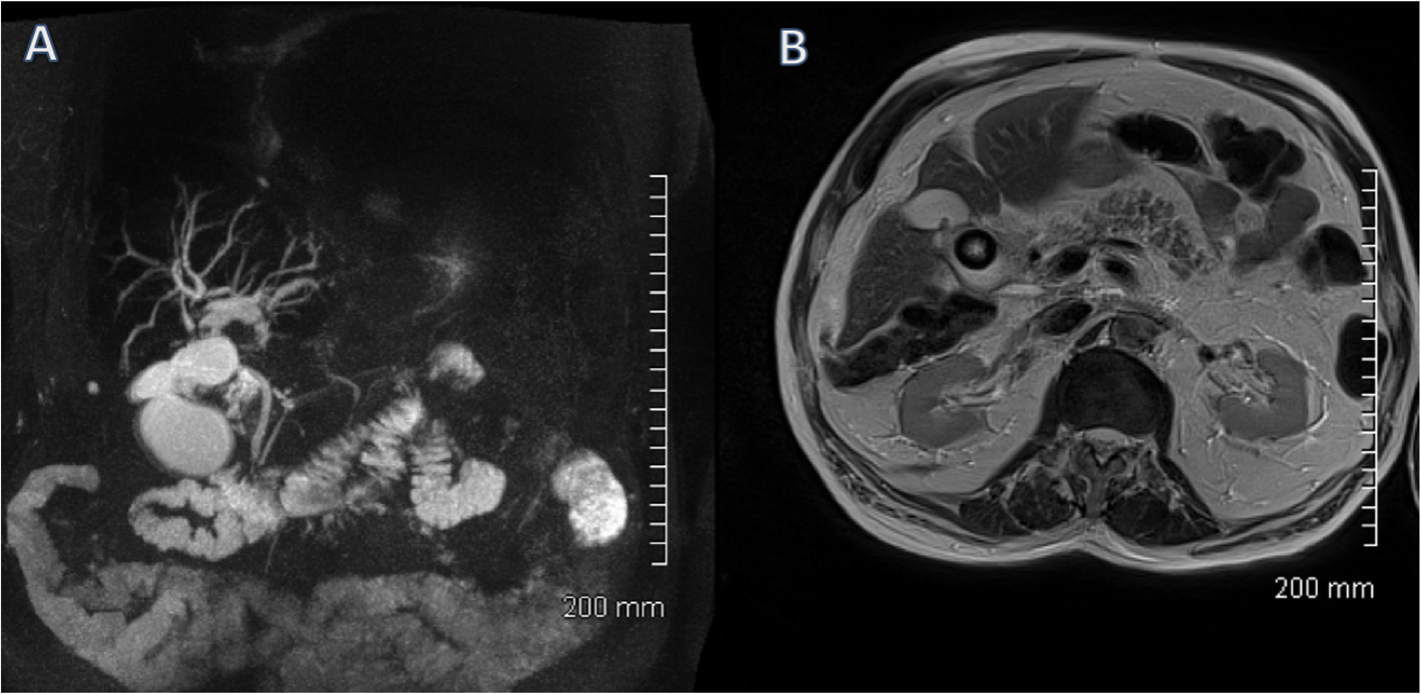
a Maximum intensity projection (MIP). b Axial T2-weighted MRCP image of the biliary tree. The gallbladder is distended with extensive intrahepatic biliary duct dilatation. The common bile duct is normal in calibre. Appearances are consistent with Mirizzi syndrome, with a stone in Hartmann’s pouch of the gallbladder causing extrinsic compression of the common hepatic duct
